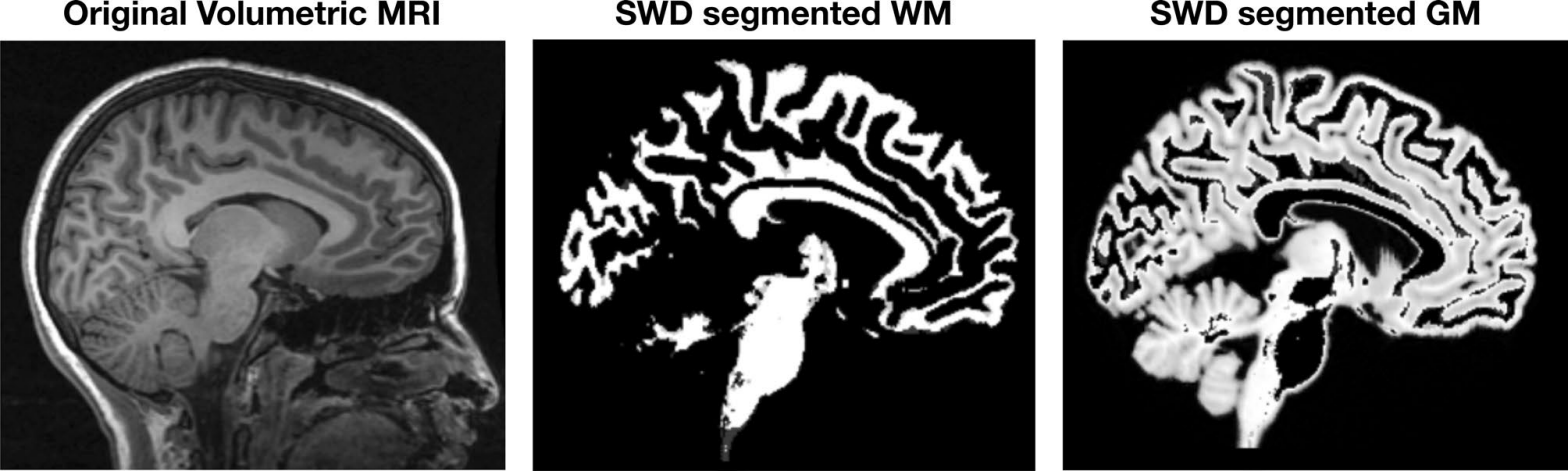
Automated human brain tissue segmentation using the Spherical Wave Decomposition (SWD) on a high resolution anatomical MRI scan. (A) Original volumetric MRI scan, (B) segmented white matter (WM) and (C) gray matter (GM) (adapted from^42^). Each of these segmented tissues are individually described by their own SWD, and thus can be used for quantative comparative analysis.
